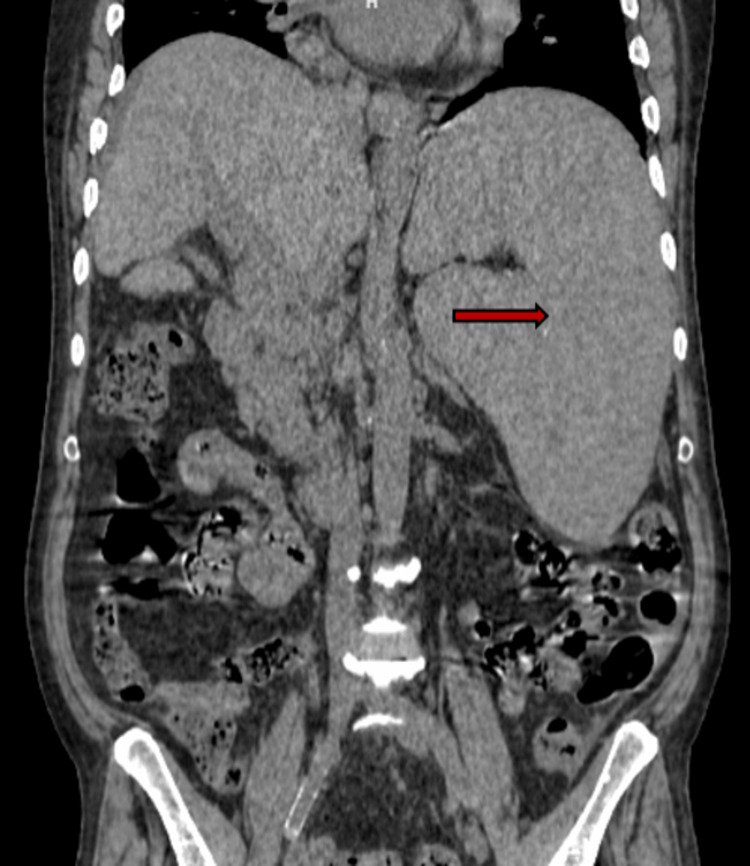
Non-contrast CT scan of the abdomen showing gross splenomegaly The red arrow shows the enlarged spleen.
